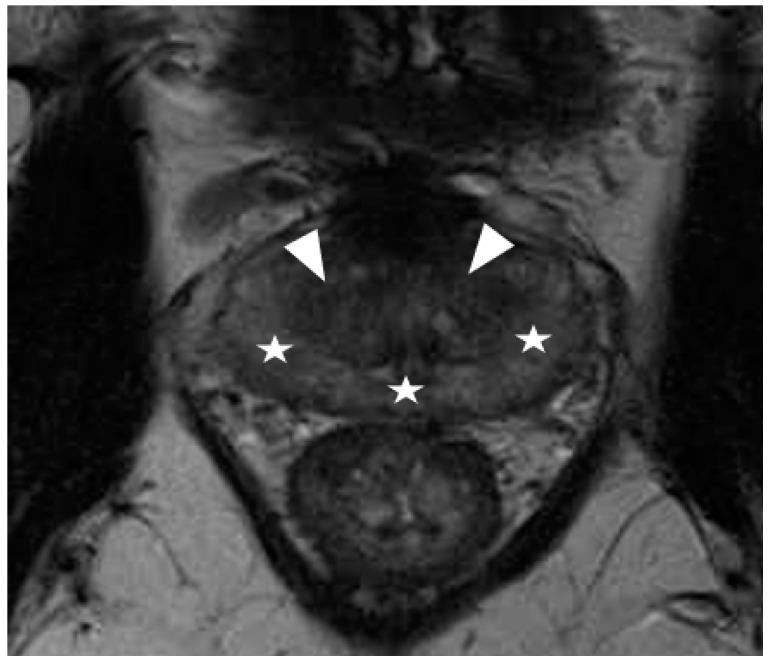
Prostatitis typically appears as diffuse hypointensity in the peripheral zone on T2-weighted imaging (star), resulting in an almost similar signal to stromal nodules related to benign prostatic hyperplasia in the transition zone (arrowhead). This may make differentiation between peripheral and transition zone difficult, and zonal segmentation challenging.
